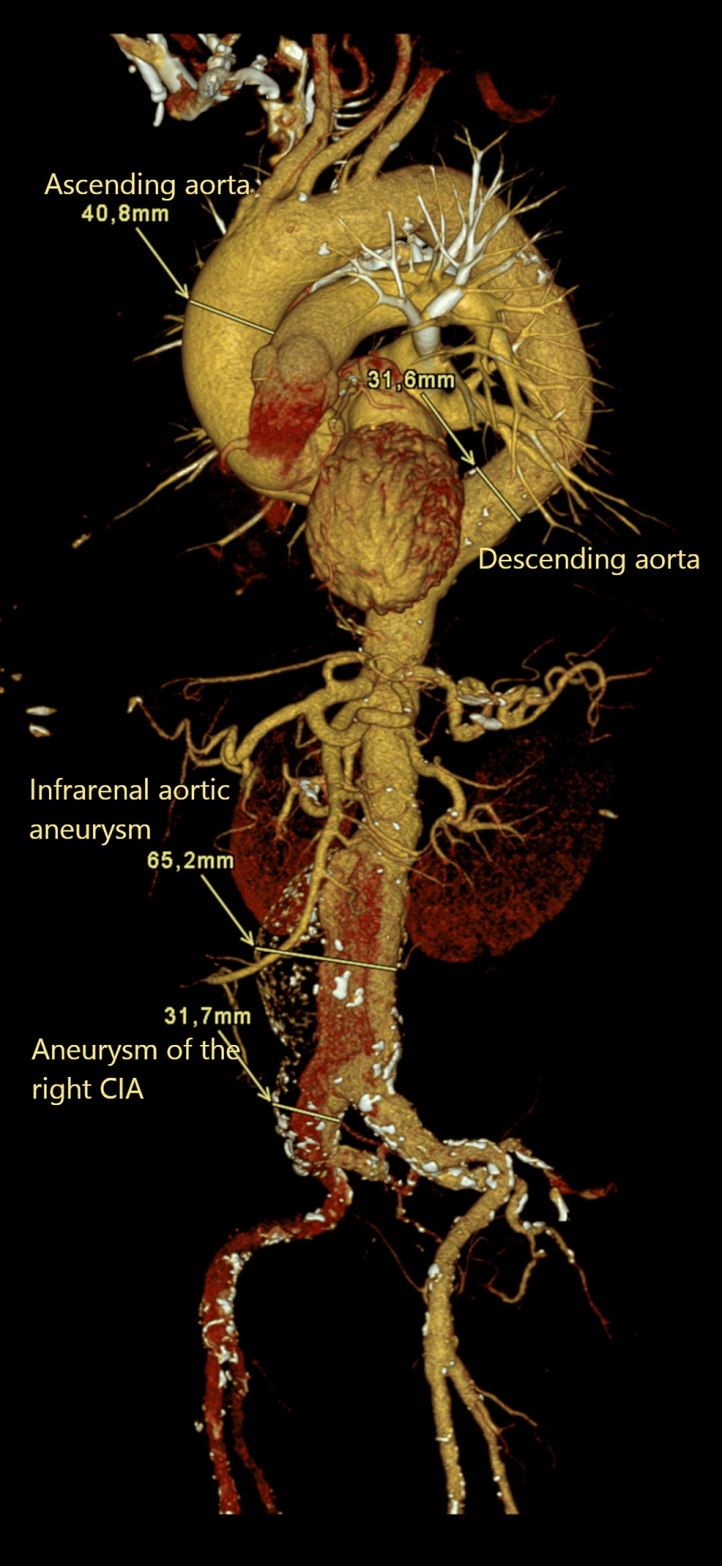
Computed tomography angiography of the initial aortic aneurysms, three-dimensional view.
